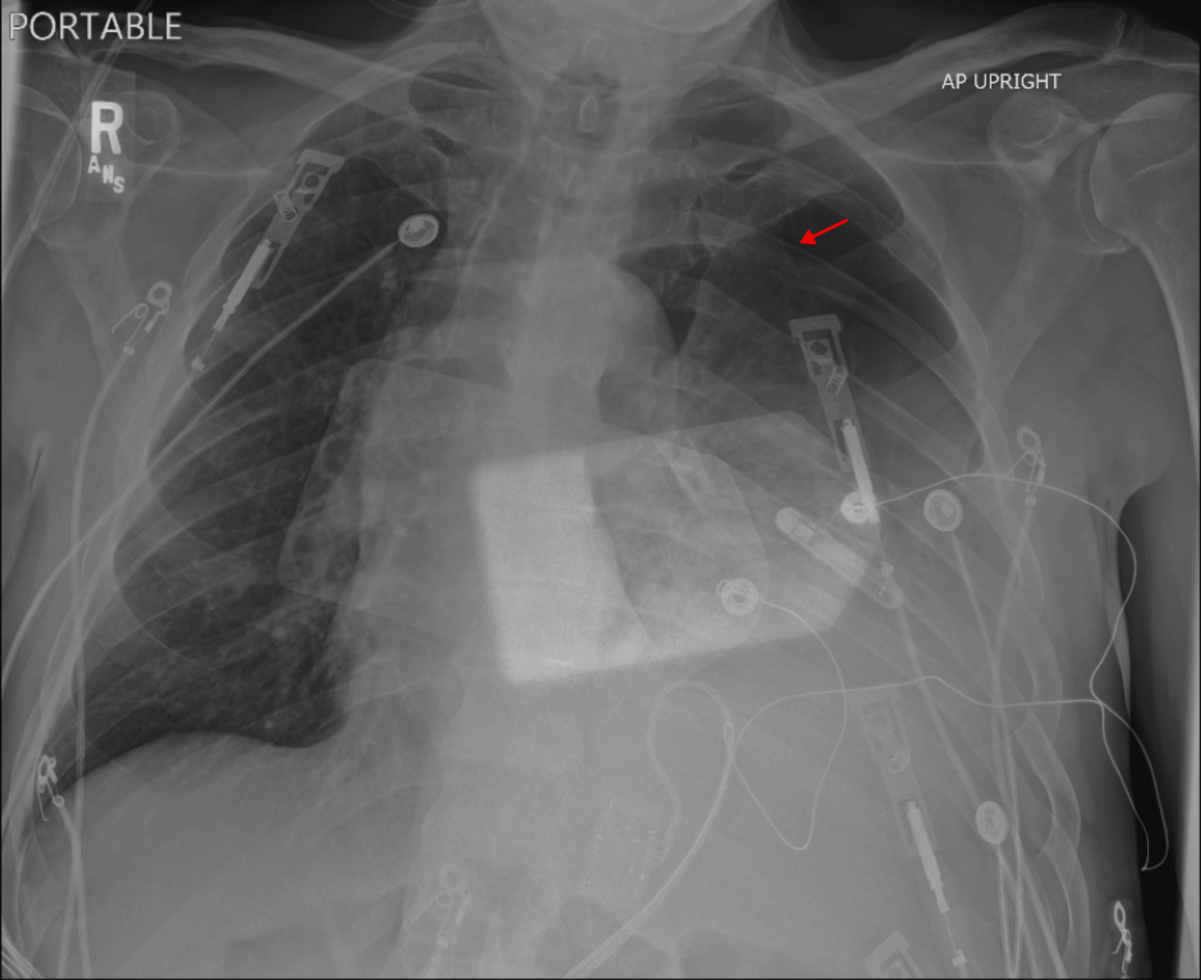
Chest radiography at initial presentation Initial chest radiography showing left-sided hydropneumothorax (arrow) with rightward displacement of the trachea. Overlying defibrillation pads are seen.
